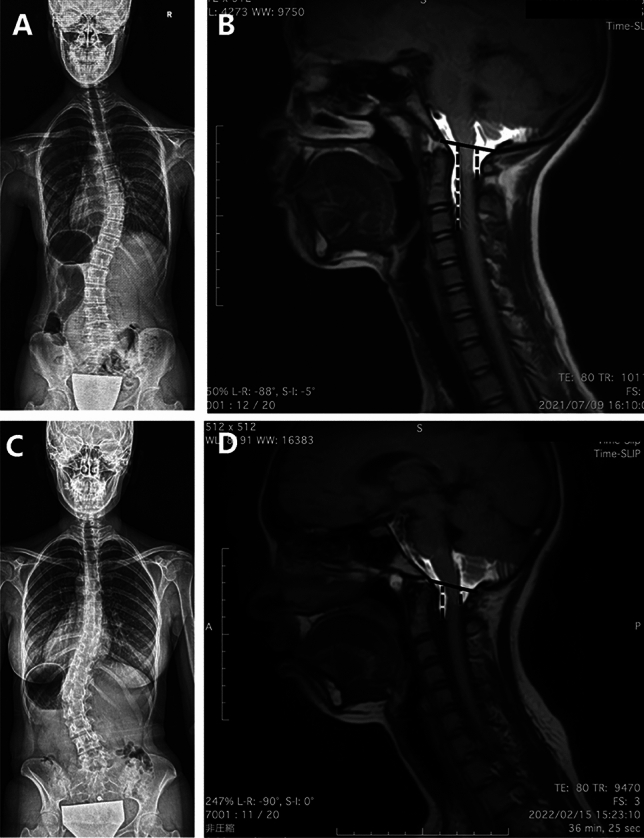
Representative Time-SLIP MRI images of Lenke type 1 and type 5 AIS patients. (A) Standing whole spine radiograph of 14-year-old female Lenke type 1 patient. Cobb angle of the major curve was 33 degrees and the Risser grade was 4. (B) Time-SLIP MRI of 14-year-old Lenke type 1 patient. The TD was 37.3 mm. (C) Standing whole spine radiograph of 19-year-old female Lenke type 5 patient. Cobb angle of the major curve was 39 degrees and the Risser grade was 5. (D) Time-SLIP MRI of 19-year-old Lenke type 5 patient. The TD was 6.3 mm.
